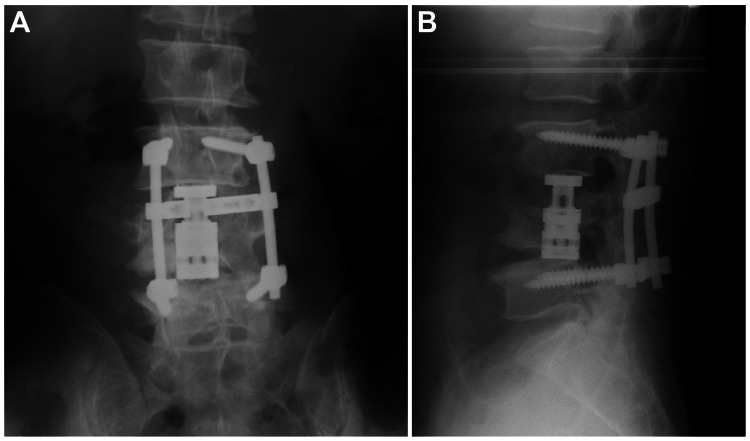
Radiographs (A: anteroposterior and B: lateral) after posterior and anterior fixation from L3 to L5.
